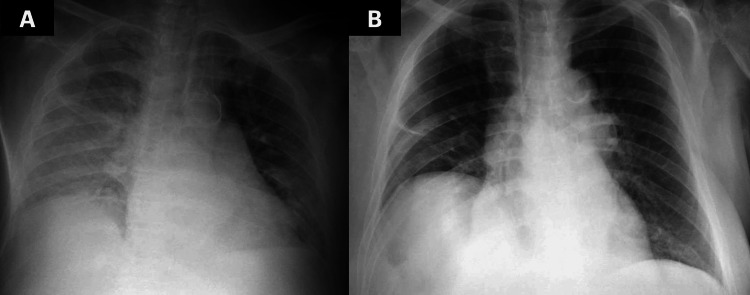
Chest radiographs from admission (A) and fifth day of hospitalization (B)
